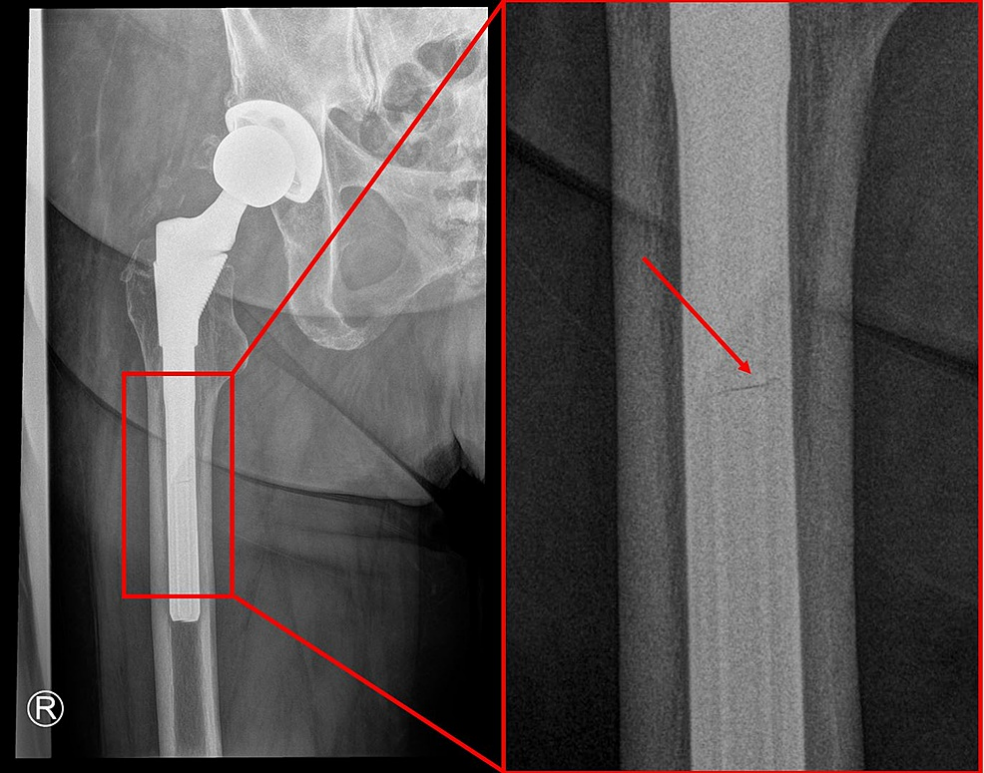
Magnified view of the preoperative anterior-posterior pelvic radiograph The magnified anterior-posterior pelvic radiograph appeared to demonstrate a small linear radiolucency thought to represent a partial fracture of the S-ROM diaphyseal stem S-ROM: Sivash-range of motion
